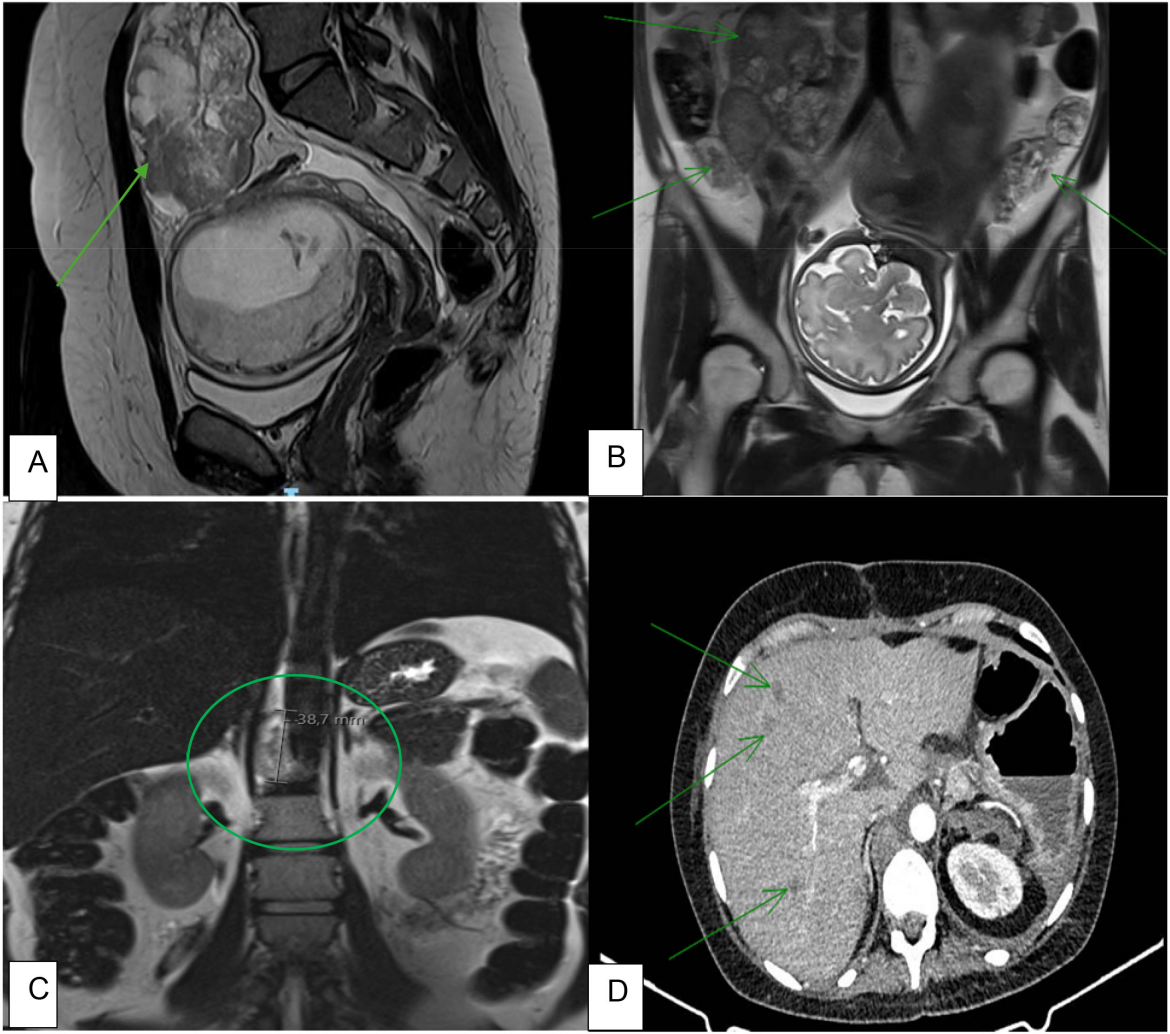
Imaging highlighting different stages of disease. (A) MRI performed during the first trimester of pregnancy showing a viable pregnancy and an ovarian tumor classified as ORADS-4. (B) MRI performed after the second cycle of carboplatin and paclitaxel, highlighting peritoneal carcinomatosis (arrow). (C) MRI performed after the second cycle of carboplatin and paclitaxel showing a retrocrural adenopathy, compatible with disease progression. (D) CT scan performed 3 weeks after surgery, showing evidence of disease progression and liver metastases (arrow).
